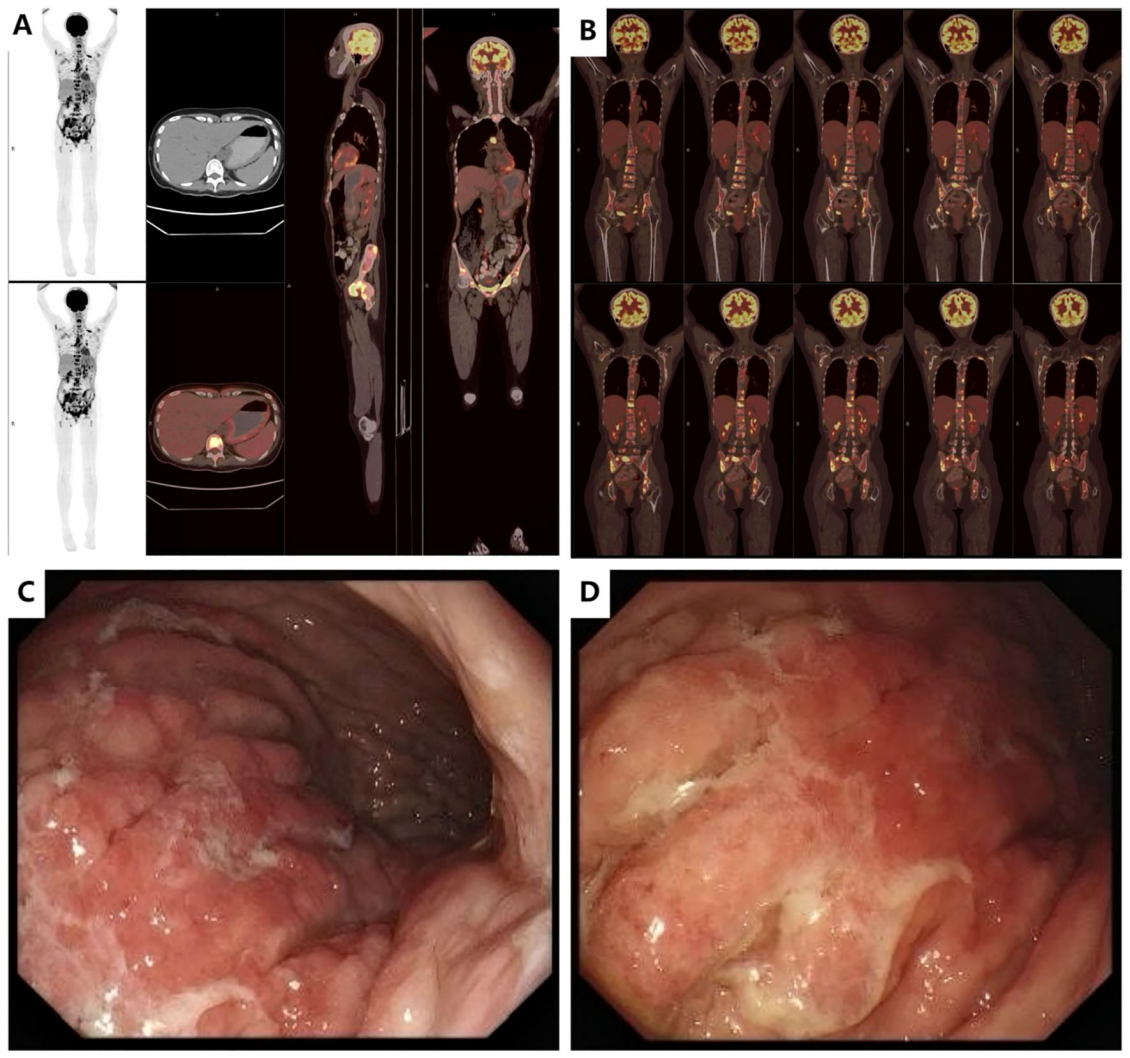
Imaging data at initial diagnosis before treatment (A). PET-CT (2023-12-11): Diffusely increased metabolic activity in the gastric fundus and body with irregular wall thickening. (B). PET-CT (2023-12-11): Multiple osteolytic/osteoblastic bone metastases involving the cervical, thoracic, and lumbar spine, sacrum and coccyx, sternum, bilateral scapulae, bilateral ribs, pelvic bones, and proximal femurs bilaterally. (C, D). Endoscopic Ultrasound (EUS, 2023-12-11): Diffuse mucosal thickening with heterogeneous hypoechoic texture. Loss of distinct submucosal layer and partial invasion into the muscularis propria layer. Maximum wall thickness: 7.28 mm. Highly suggestive of linitis plastica (leather bottle stomach).
